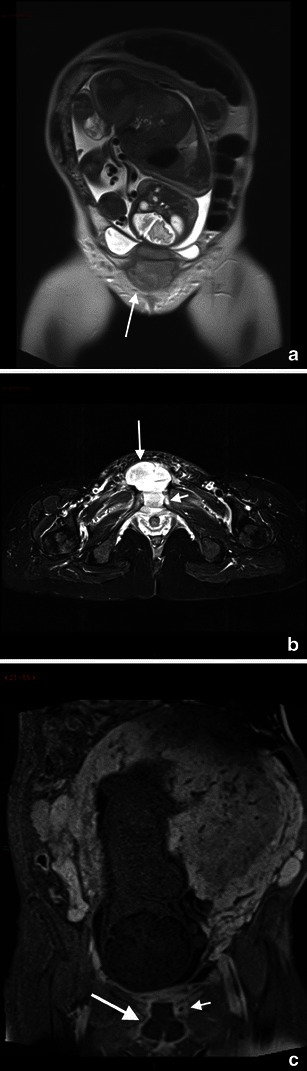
A 35-year-old woman presents with pubic pain, fever and elevated inflammatory markers. Coronal T2–weighted HASTE image (a) and T2–weighted STIR image (b) show fluid collection (arrow) at the level of the symphisis pubic bone with hyperintensity of the pubic bone (short arrow). Contrast T1-weighted image shows the presence of a thick vascularised wall indicative of an abscess with a small abscess at the level of left pubic bone (short arrow). These findings are in agreement with osteomyelitis
